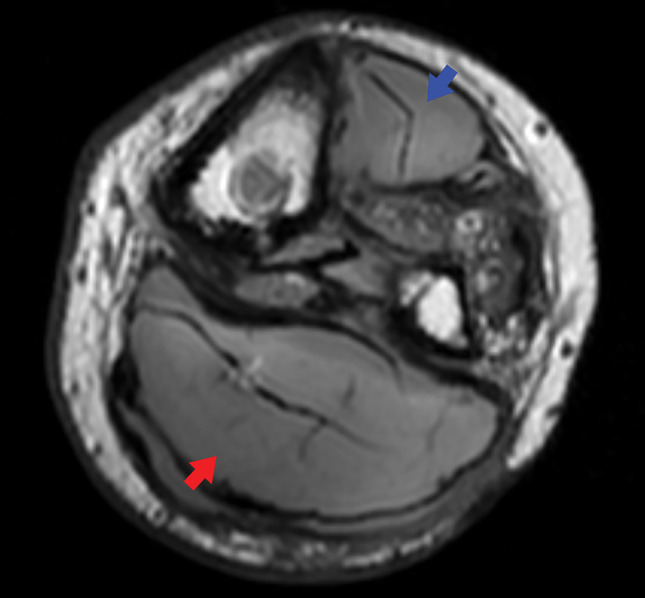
Axial MRI of the left lower leg at the initial presentation. Necrotic changes are observed in the tibialis anterior muscle (blue arrowhead) and the triceps surae (gastrocnemius–soleus) muscle (red arrowhead).
